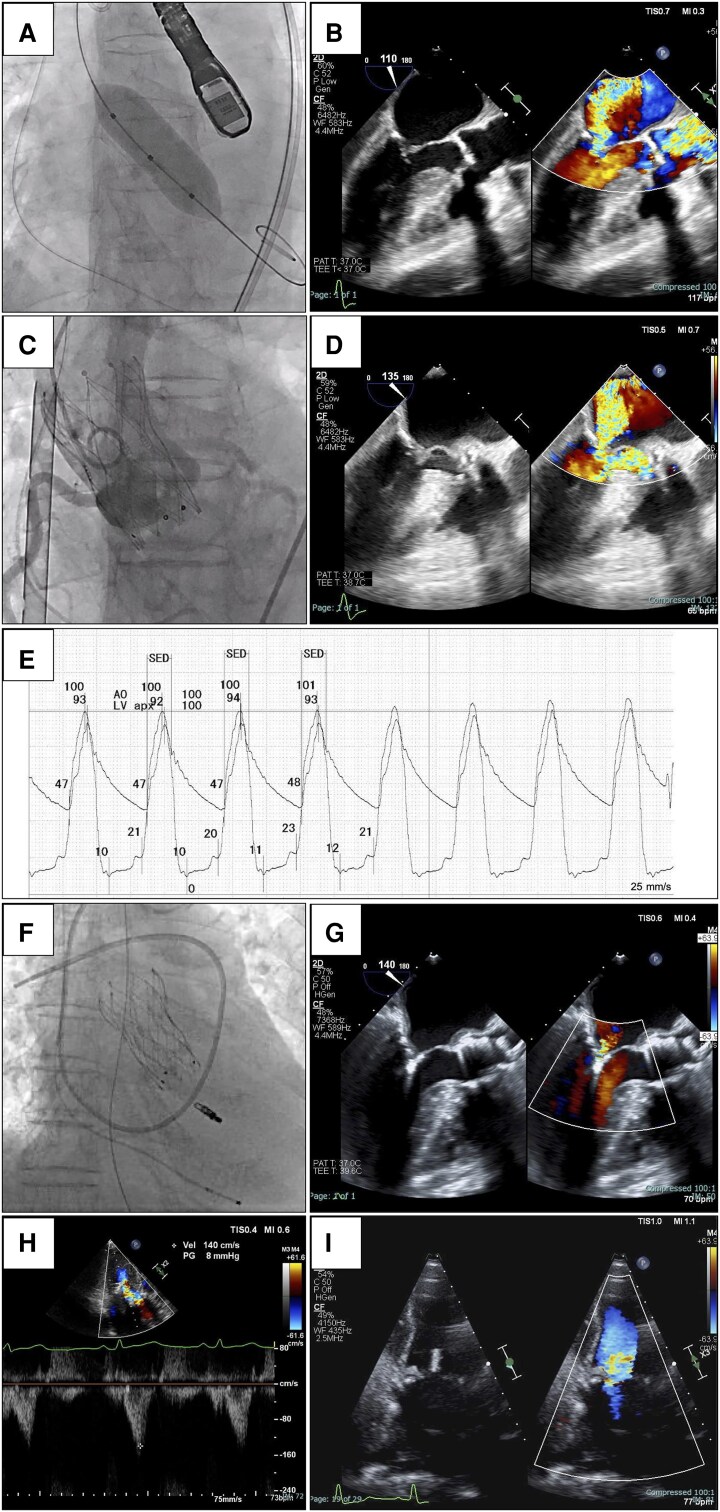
Angiography and transoesophageal echocardiographic findings during the periprocedural course. (*A*) Fluoroscopic image during balloon predilatation using an 18-mm balloon. (*B*) Transoesophageal echocardiography image immediately after predilatation showing mitral leaflet malcoaptation and severe mitral regurgitation. (*C*) Aortography after implantation of a 25-mm Navitor valve. (*D*) Severe mitral regurgitation (MR) persisting after valve deployment. (*E*) Simultaneous pressure measurement before mitral transcatheter edge-to-edge repair (M-TEER) shows no significant left ventricular outflow tract (LVOT) pressure gradient. (*F*) After deployment of MitraClip NT at the central A2–P2 segment. (*G*) After M-TEER, the MR improved from severe to mild. (*H*) LVOT flow acceleration is not observed after M-TEER. (*I*) Follow-up echocardiography image showing mild residual mitral regurgitation. Abbreviations: LVOT, left ventricular outflow tract; MR, mitral regurgitation; M-TEER, mitral transcatheter edge-to-edge repair; TAVI, transcatheter aortic valve implantation.
